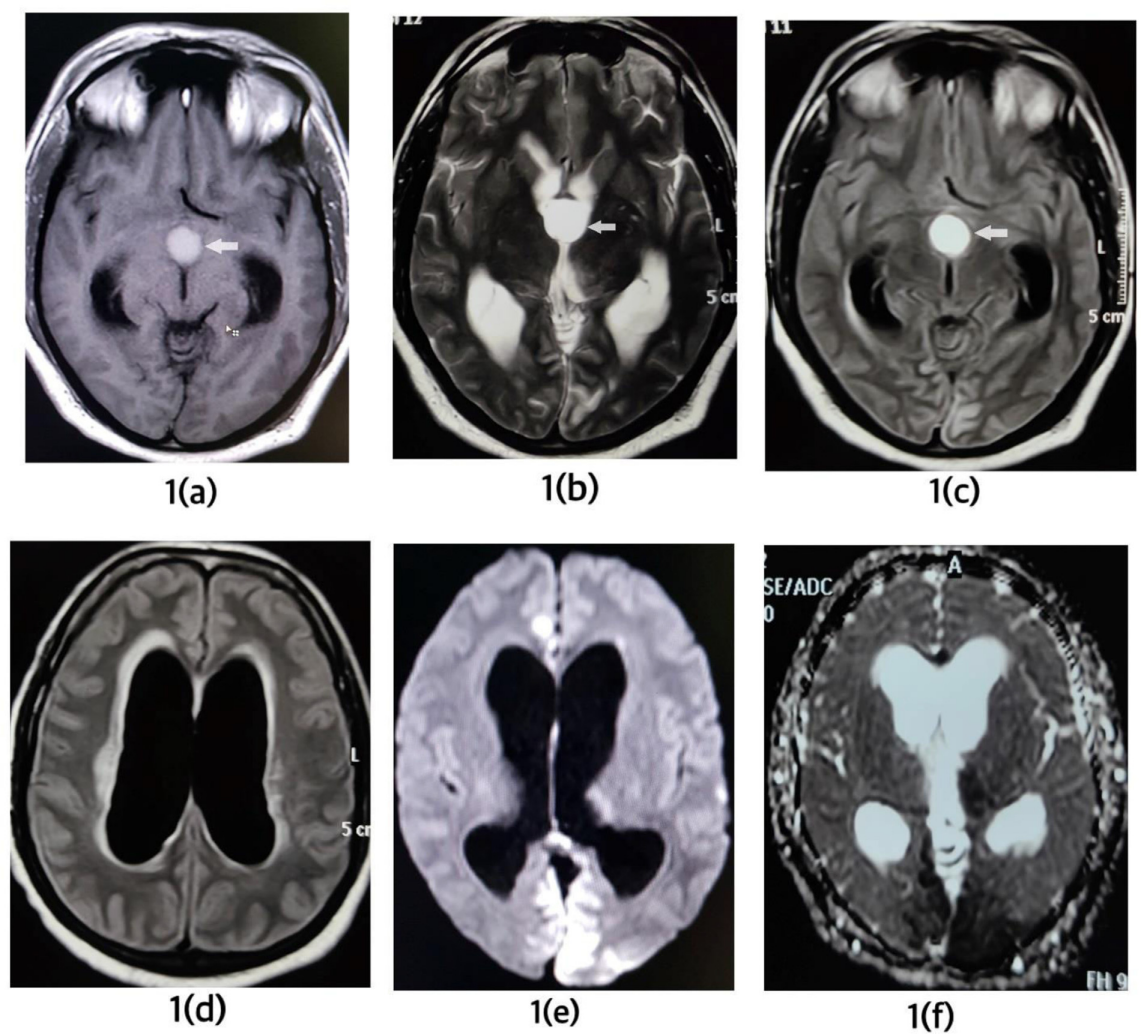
(A and B) are T1- and T2-weighted MRI images showing high signal intensity cyst (pointed by an arrow) in third ventricle. (C) is FLAIR image showing high signal of colloid cyst. (D) is fluid attenuated inversion recovery (FLAIR) image showing active hydrocephalus with mild periventricular ooze. (E) is diffusion-weighted image and (F) is ADC image showing infarcts in bilateral occipital lobes and left thalamus. There were infarcts in left part of midbrain, pons, left thalamus, and left superior cerebellum. CC did not show diffusion restriction.
